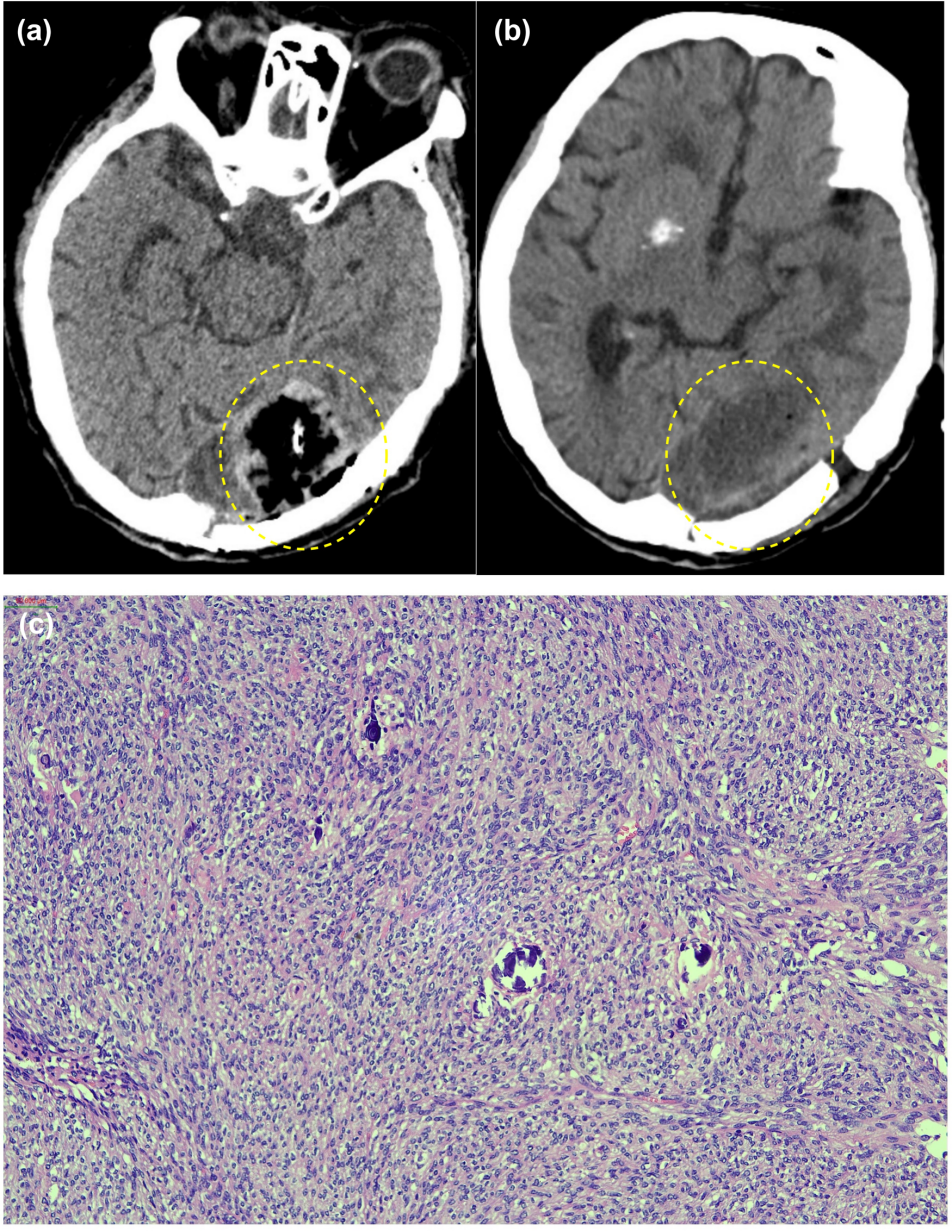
Postoperative pathology and imaging. No residual tumor was observed within the yellow dashed circle. Panel (a) displays the head CT on the second day postoperatively, while panel (b) displays it on the eighth day. The operation field is clean, and there is no intracranial bleeding. Panel (c) presents the pathology of a Grade 1 fibrous meningioma, with a Ki-67 index of 1%.
